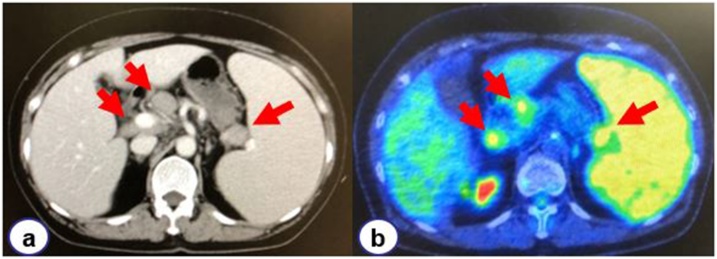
Computed tomography and PET findings. A splenomegaly, and multiple intraabdominal swollen lymph nodes were detected at splenic hilum, hepatoduodenal ligament, and along common hepatic artery (a). PET showed diffusely enhanced uptake in the spleen, intraabdominal lymph nodes and splenic hilum (b).
